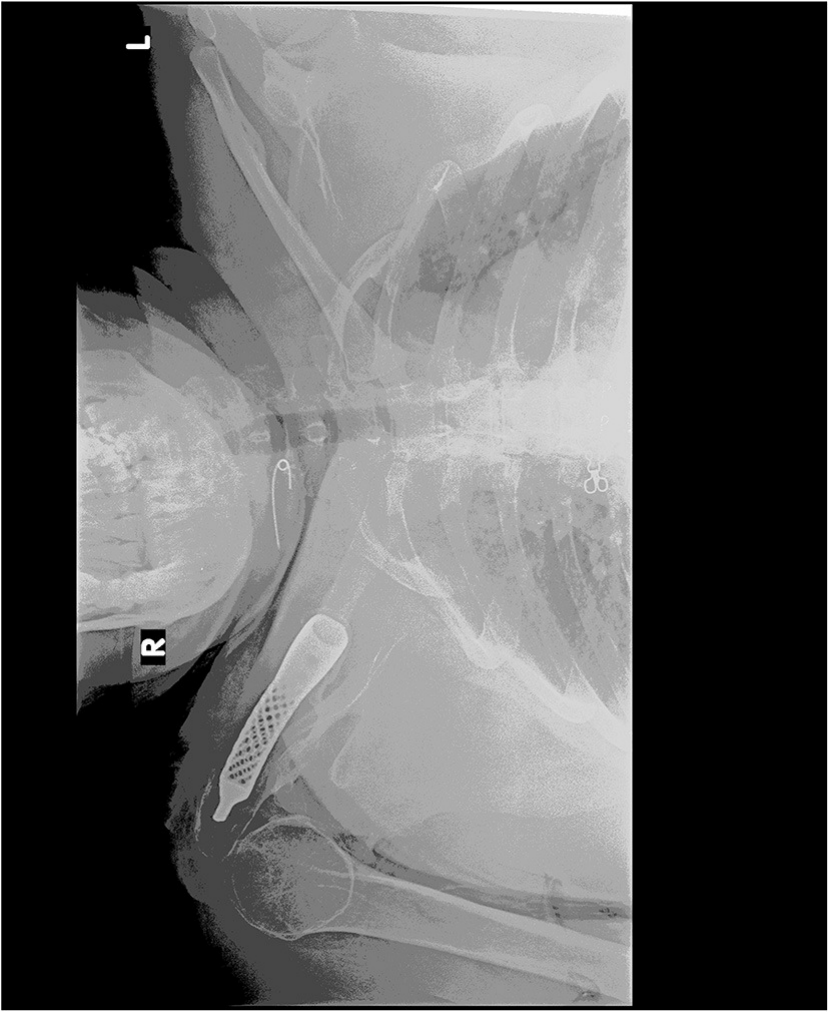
Postoperative radiograph at 2-year follow-up revealed a good position of the prosthesis.
